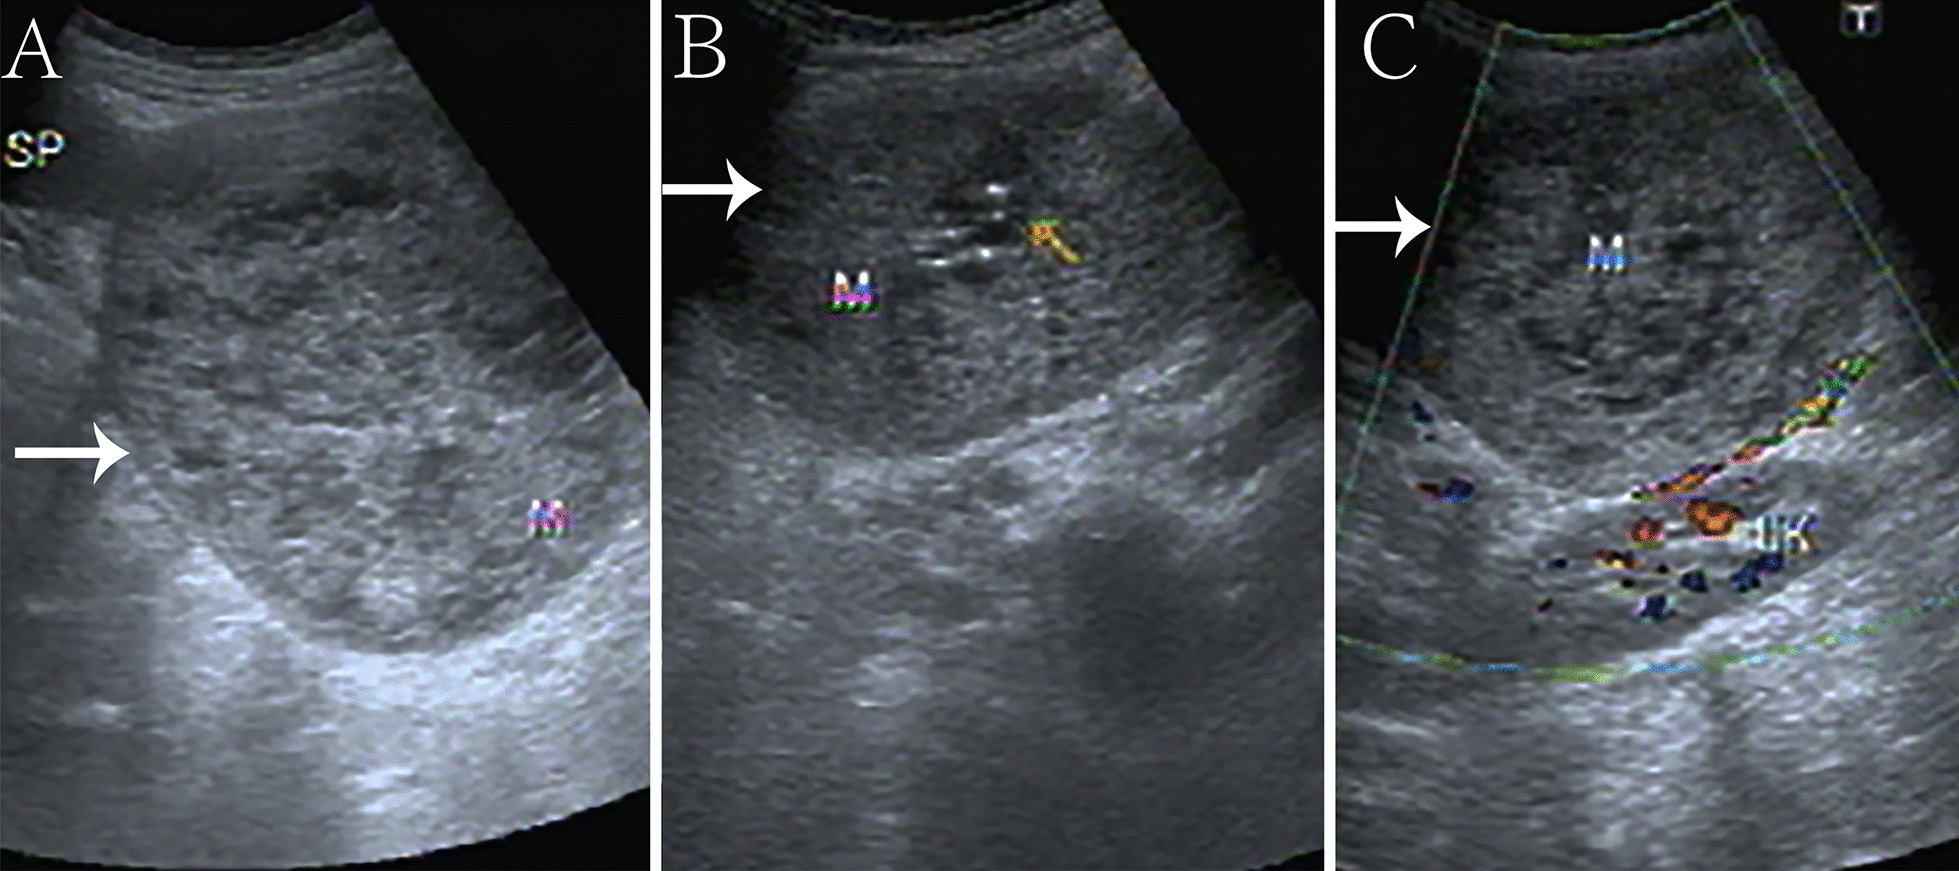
Grayscale and Doppler ultrasonography show a roundish heterogeneous mass below the spleen in the middle and lower abdomen on the left, with well defined margins, heterogeneous echotexture, with punctate echogenic foci, no acoustic shadowing (A, B). Doppler revealed multiple foci of vascularity within the mass, which also displayed peripheral vascularity (C)
